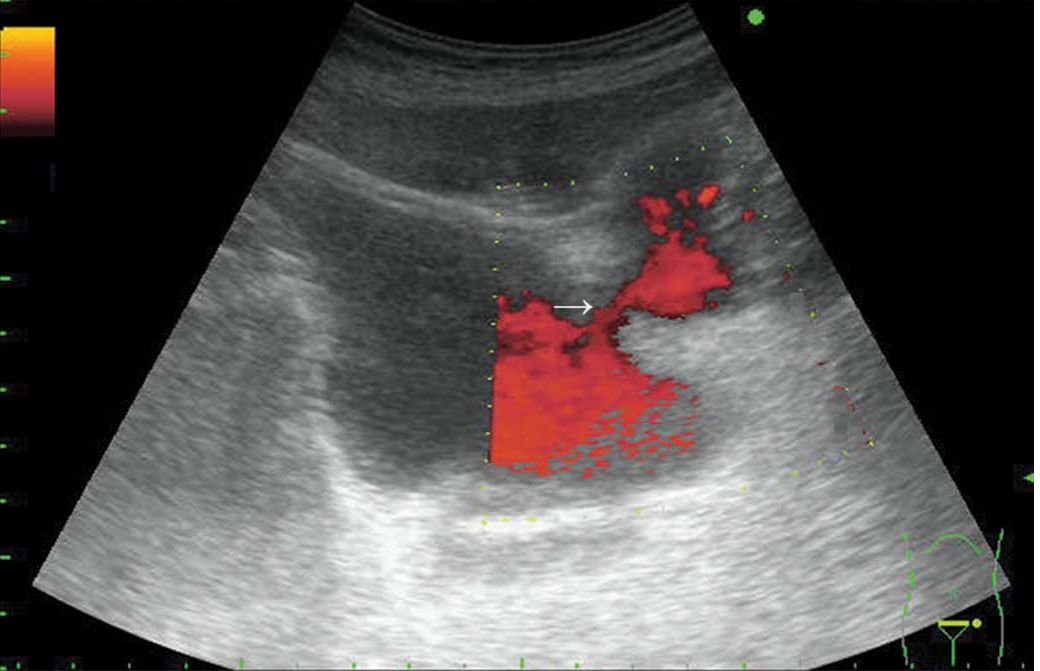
Power Doppler sonography revealing a communication (arrow) between urinary bladder and diverticulum.
